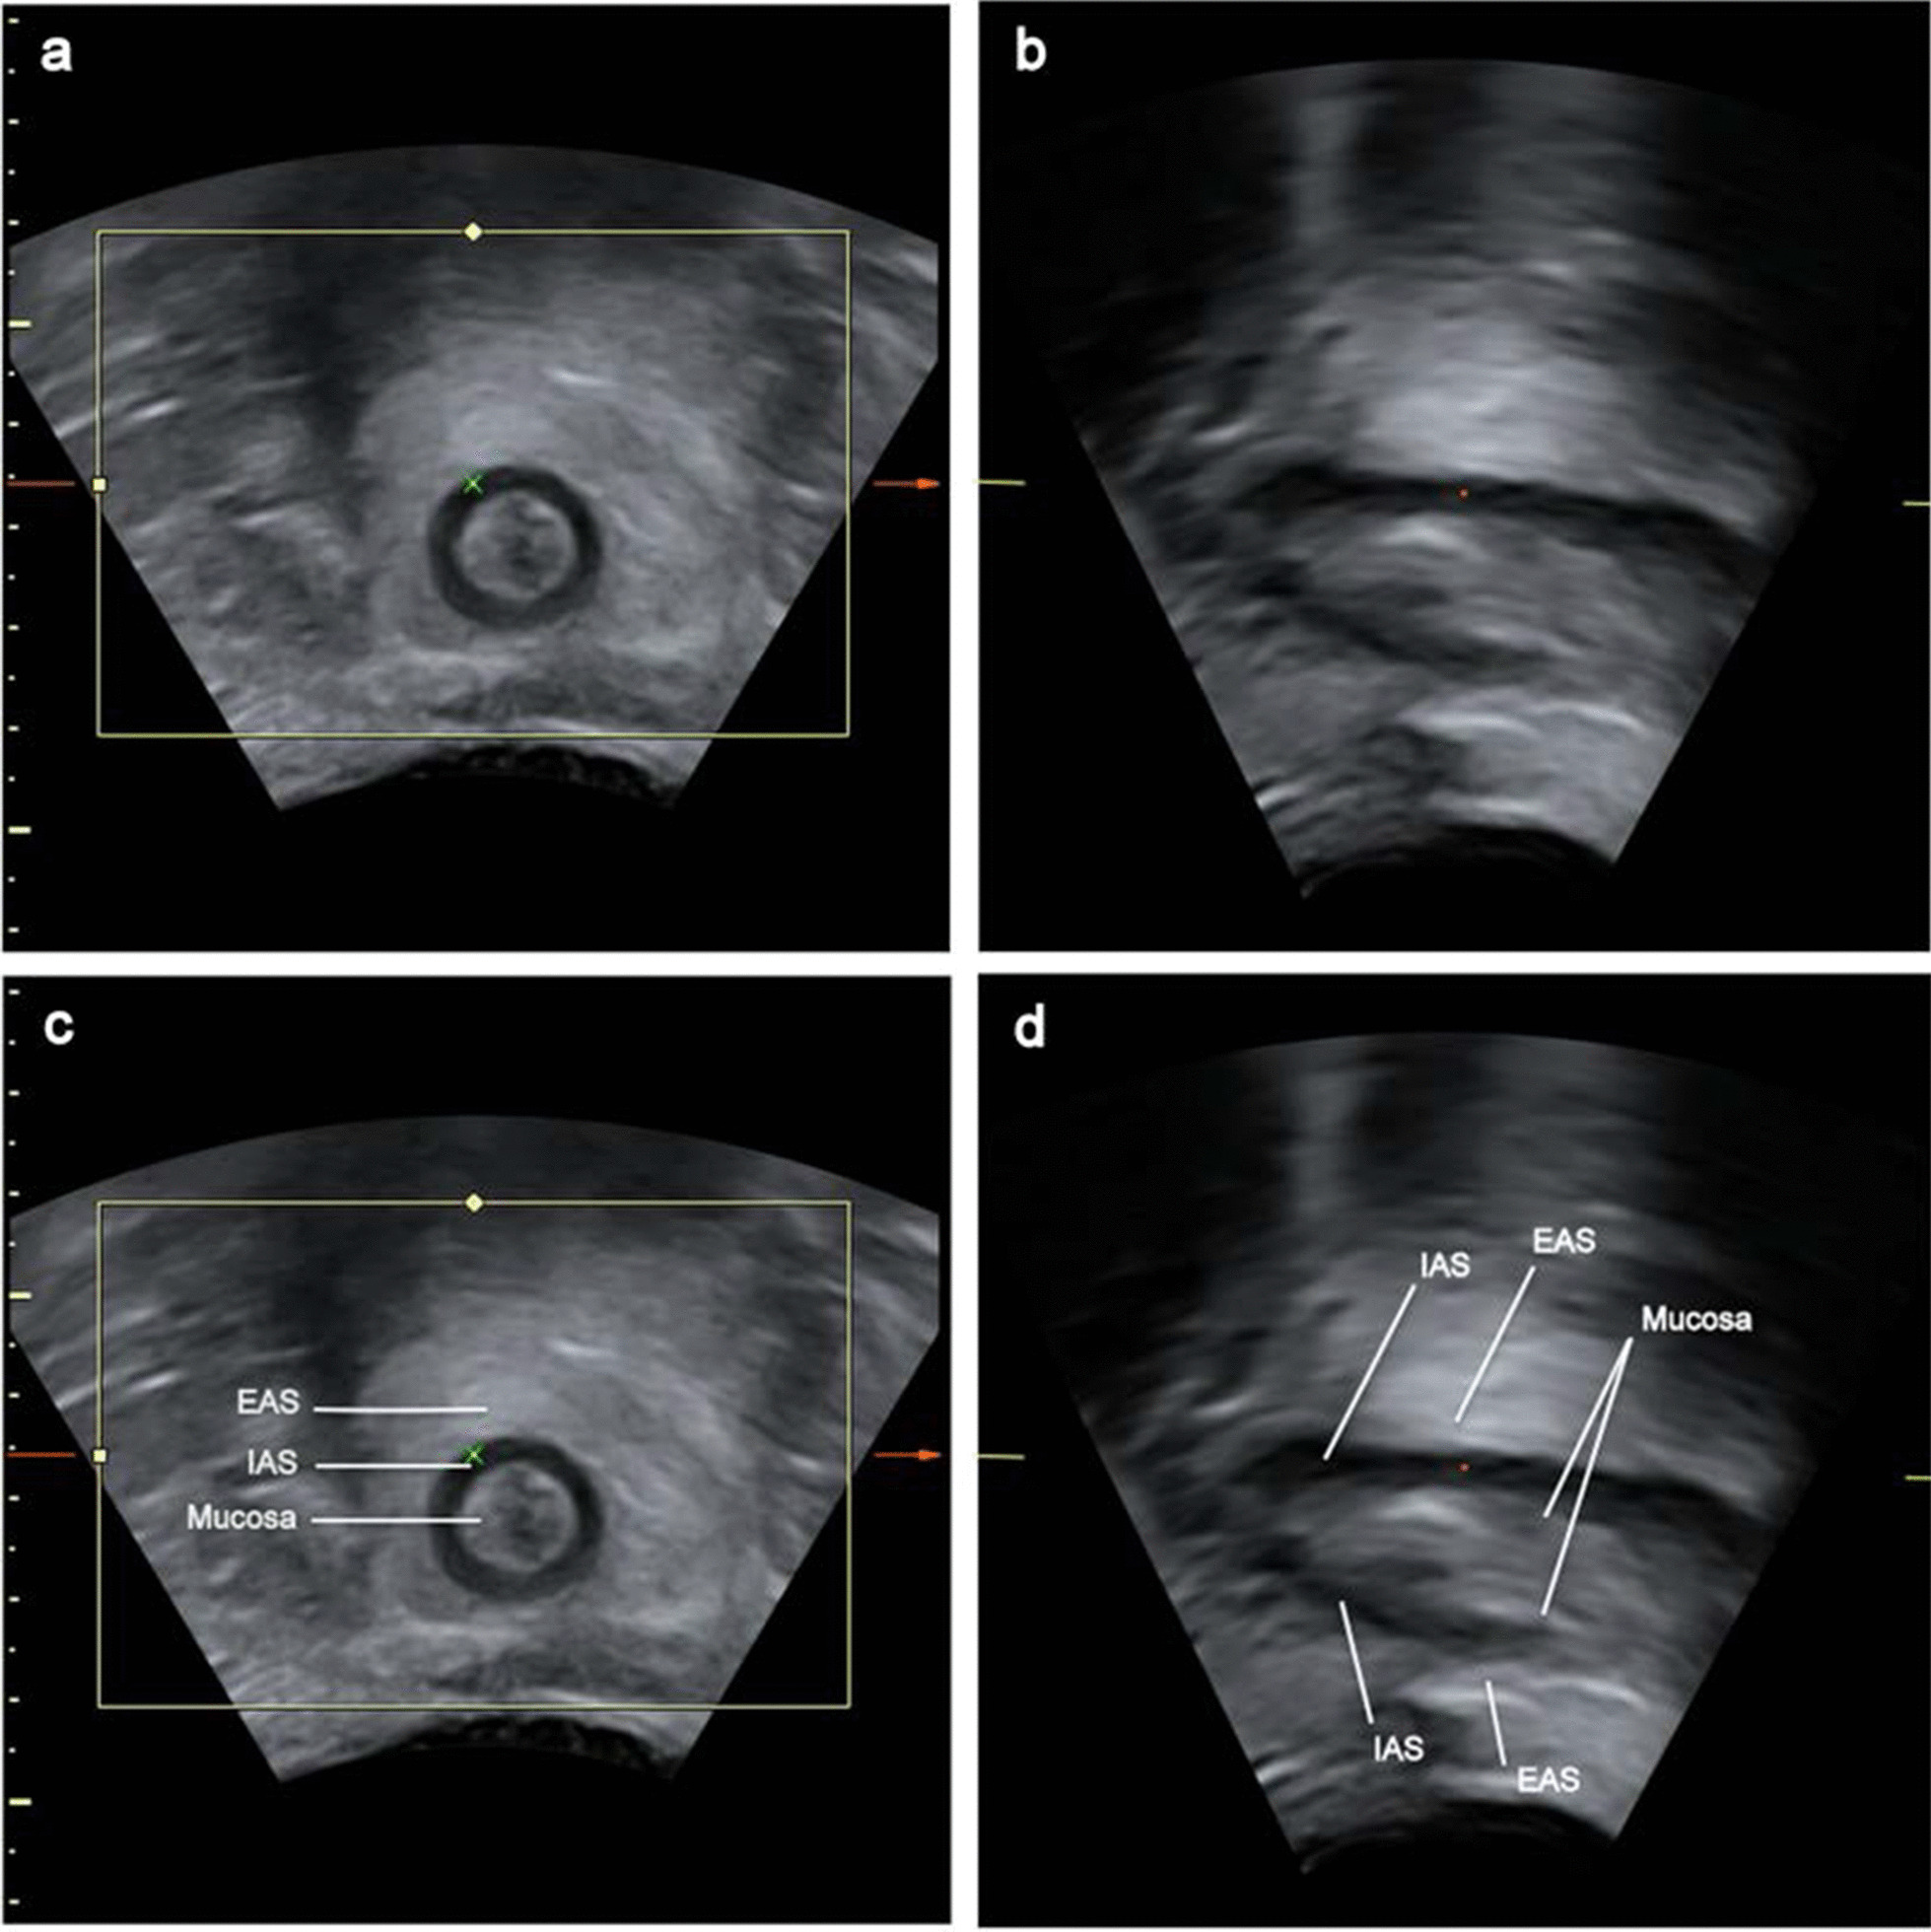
Perianal ultrasound (PAUS) of the anal canal at rest. a + c Coronal plane, b + d midsagittal plane
